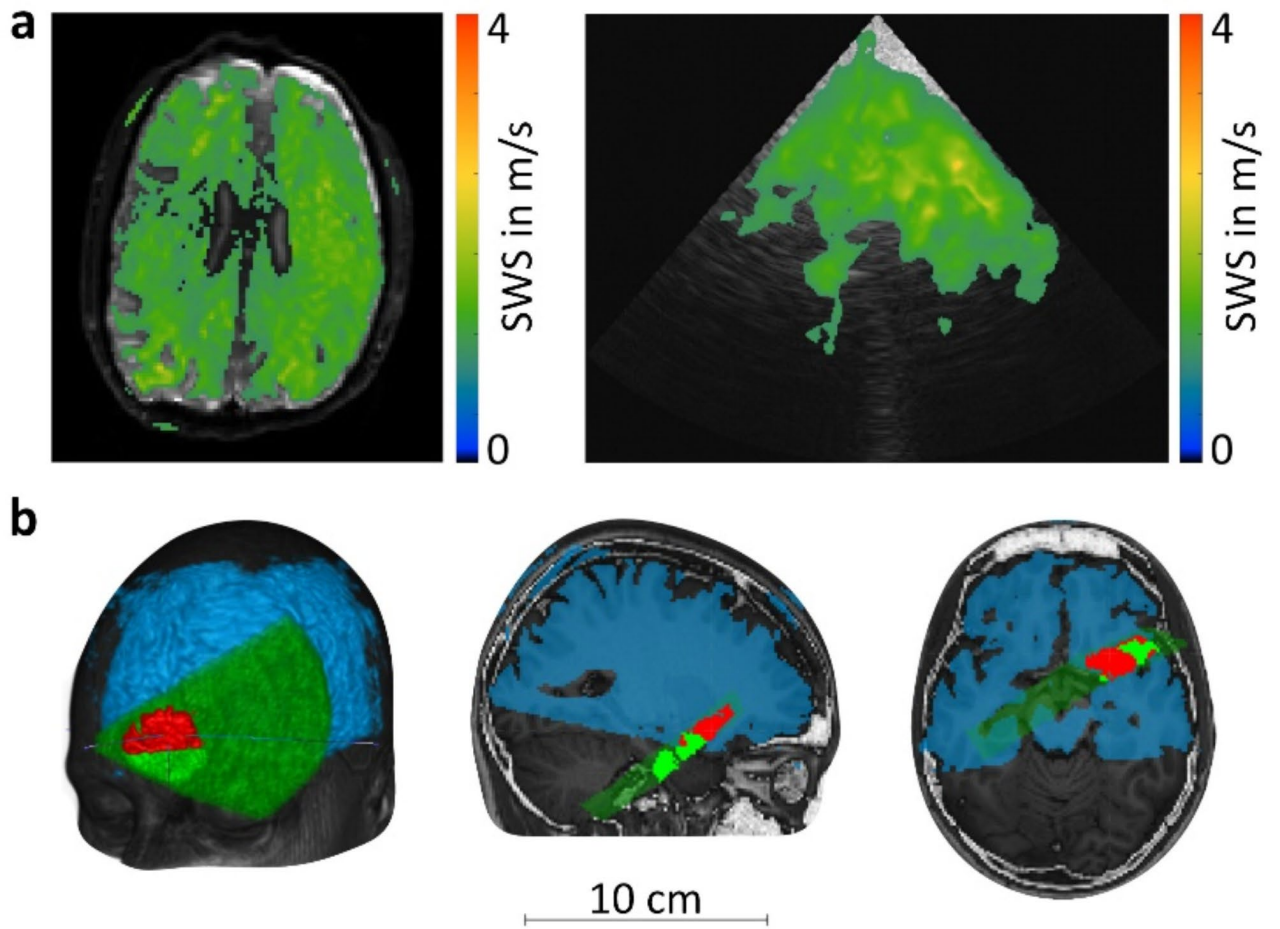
(a) Examples of thresholded elastograms generated from (left) MRE and (right) THE. (b) Three views of the temporal lobe region (red) of a subject after shear wave speed thresholding and median-averaging. This region results from the derived threshold of 0.9 m/s and the overlap of more than 8 THE scans. Gray: T1-weighted 3D MRI for anatomical orientation. Blue: thresholded MRE. Dark green: field of view of THE. Light green: thresholded THE. Red: overlap region of thresholded MRE and THE, i.e., temporal lobe region.
